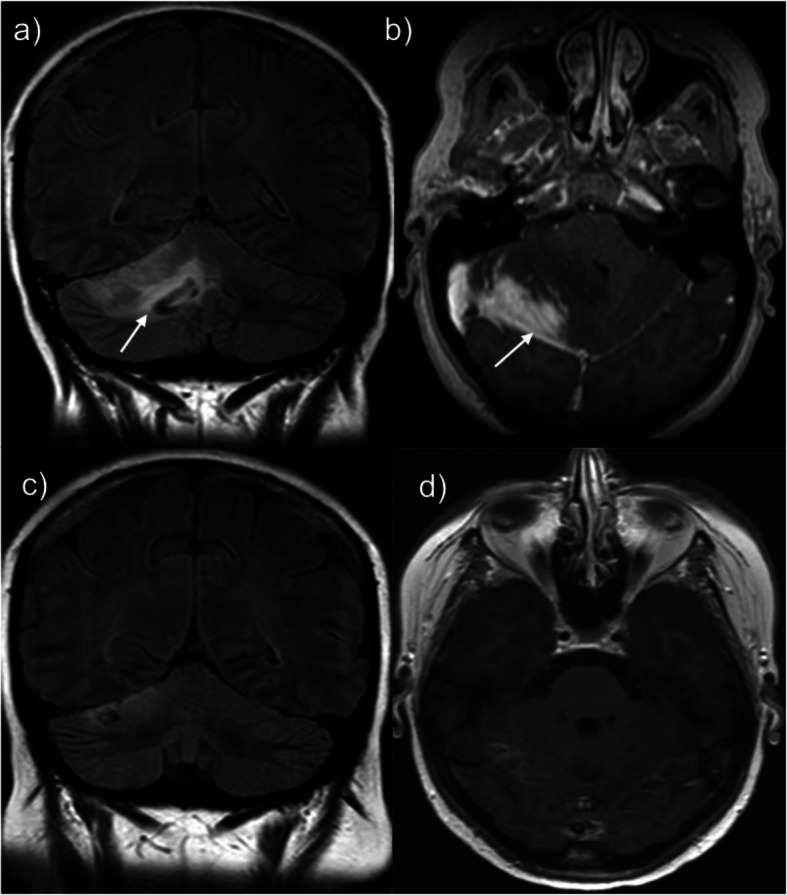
MRI brain imaging. MRI Brain showing high signal in the right cerebellar hemisphere. a Coronal FLAIR, arrow with a corresponding abnormal focal area of enhancement shown on the b Axial post-gadolinium images. Repeat MRI Brain after 3 months (while the patient was on treatment) shows almost complete resolution of these findings c Coronal FLAIR d Axial post-gadolinium
